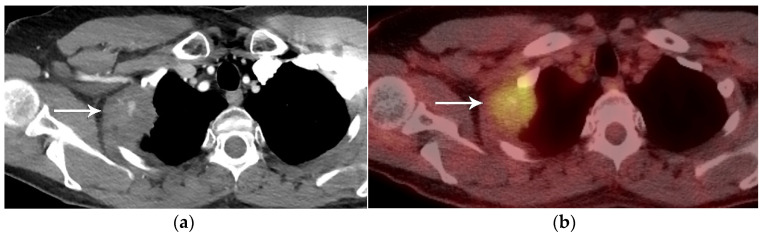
T3 disease. (a) Contrast-enhanced CT, and (b) axial PET/CT show the FDG-avid right upper lobe 4 cm primary tumor invading the chest wall (arrow). The size of 4 cm is T2a disease while chest wall invasion is T3 disease. With no nodal or distant metastases, the stage is IIB.
